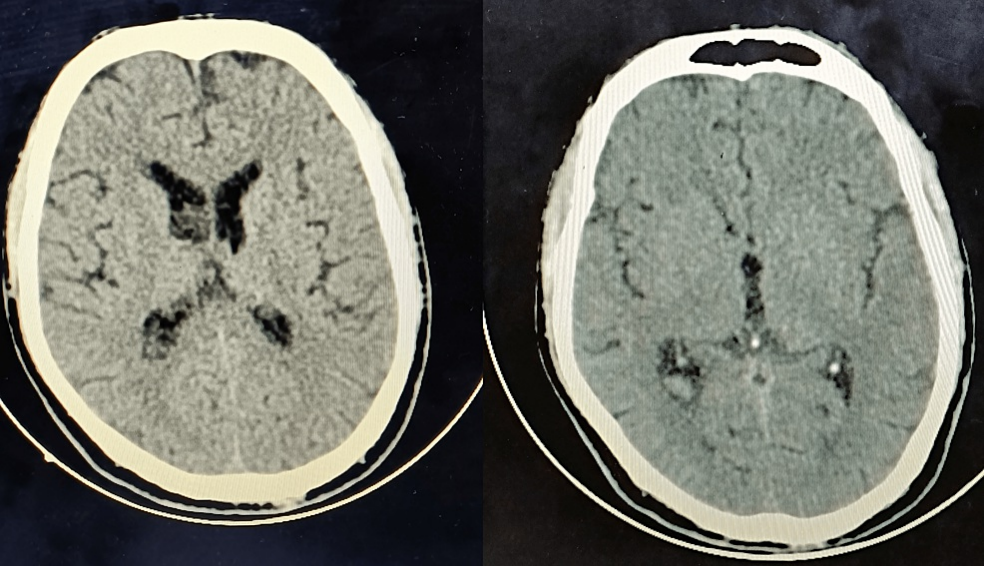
NCCT head after two weeks of the presentation showing complete resolution NCCT: non-contrast computed tomography
